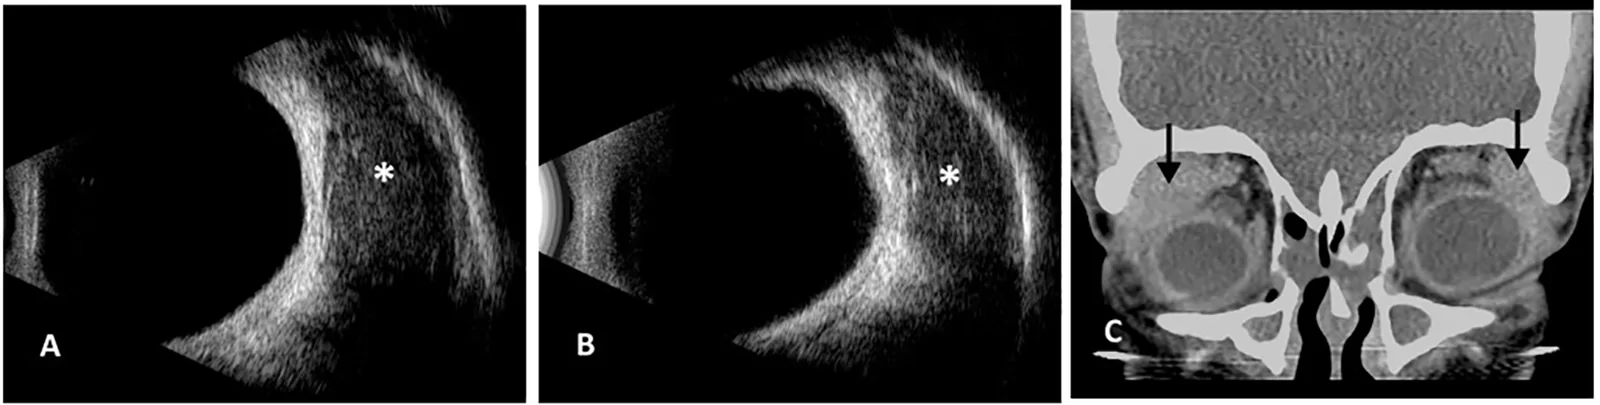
IgG4-Related Dacryoadenitis. (A, B) Longitudinal B-scan of the right eye and left eye, respectively, aimed superotemporally, show clearly outlined, oval-shaped enlargement of the lacrimal gland (asterisk) displaying a medium reflective honeycomb appearance. During the exam, the mass demonstrated slight compressibility when pressure was applied with the probe. (C) Coronal CT scan demonstrates enlarged lacrimal glands at the superotemporal aspect of the orbits (arrows). The asterisk (*) is used to label an area of the figure.
